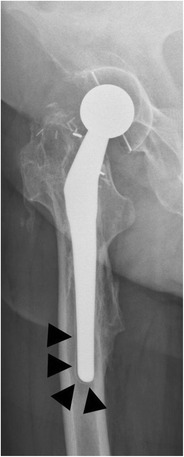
AP radiograph. Cementless total hip arthroplasty. A < 2 mm lucency, outlined by a discrete sclerotic margin, in Gruen zone 3/4 (*arrowheads*): this indicates a fibrous rather than bony ingrowth, thought to provide sufficient stability
